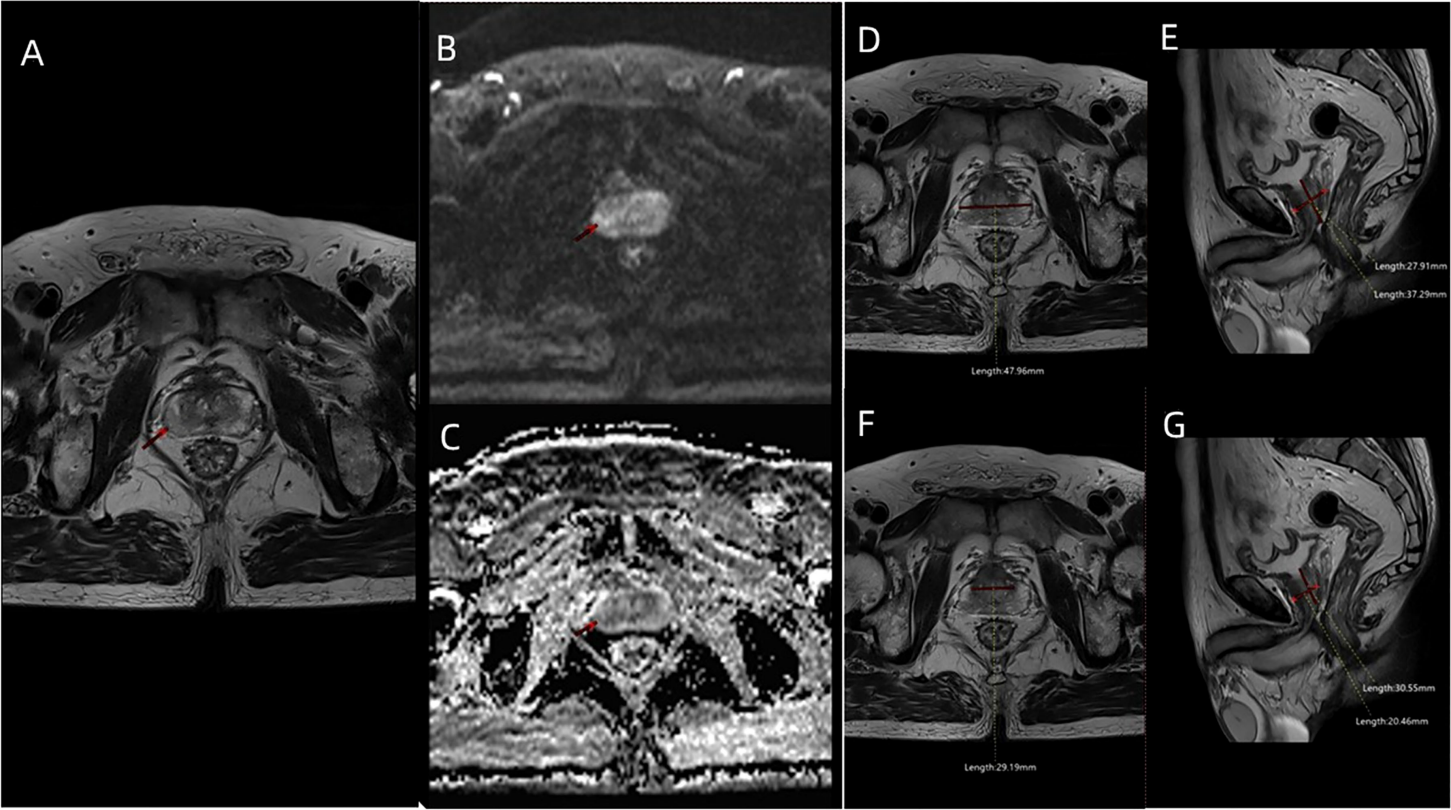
The MRI images including (A) T2WI sequence, (B) DWI sequence, and (C) ADC sequence of a patient (age: 66 years; PSA: 7.8 ng/ml; f/tPSA: 0.138; ISUP GG: 2) with PIRADS 3 ROI in the right lateral posterior of peripheral zone. (D) Maximum transverse diameter of prostate (47.96 mm) measured on axial T2WI, and (E) maximum longitudinal diameter (37.29 mm) and maximum AP diameter (27.91 mm) of prostate measured on mid-sagittal T2WI. (F) Maximum transverse diameter of transitional zone (29.19 mm) measured on axial T2WI, and (G) maximum longitudinal diameter (30.55 mm) and maximum AP diameter (20.46 mm) of transitional zone measured on mid-sagittal T2WI.
